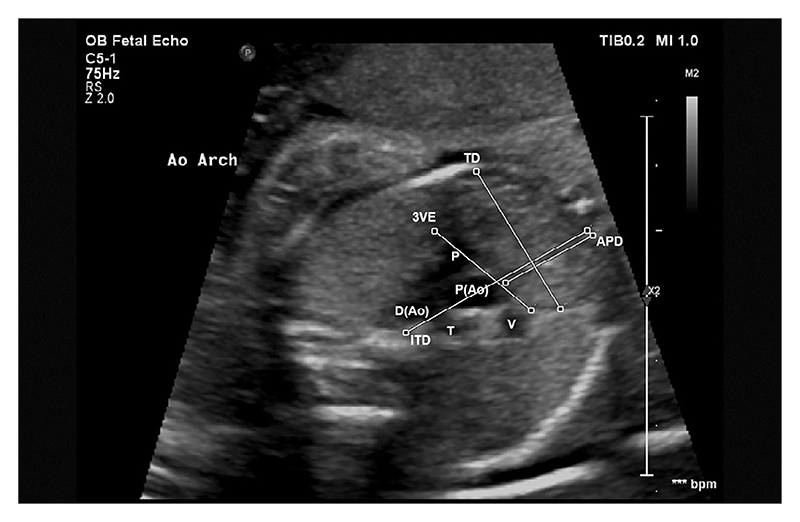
Obstetric ultrasound of fetal thorax showing the 3-vessel-trachea view (3VT). Pulmonary artery (P), proximal aorta (P(Ao)), distal aorta (D(Ao)), superior vena cava (V), trachea (T), Anterior-Posterior Diameter (APD), Intrathoracic Diameter (ITD), Transverse Diameter (TD) and 3-vessel-edge (3VE).
